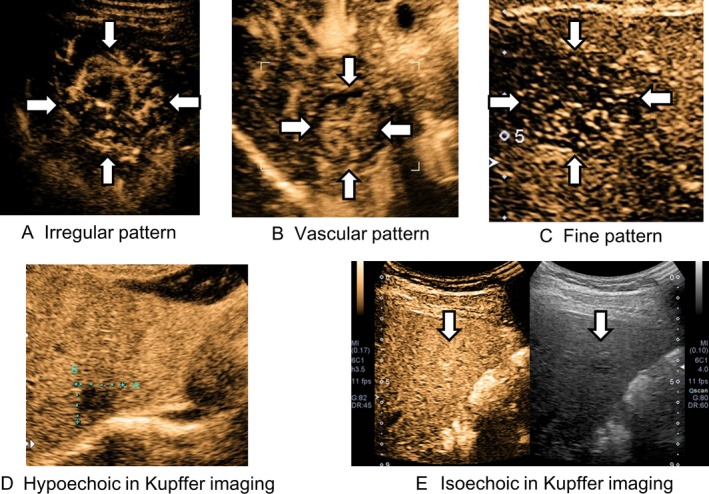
Images of MIP classification and Kupffer imaging. (A) Irregular pattern in MIP classification. (B) Vascular pattern in MIP classification. (C) Fine pattern in MIP classification. (D) Hypoechoic pattern in the Kupffer imaging in CEUS with Sonazoid. (E) Isoechoic pattern in the Kupffer imaging in CEUS with Sonazoid. MIP, maximum intensity projection; CEUS, contrast‐enhanced ultrasonography.
